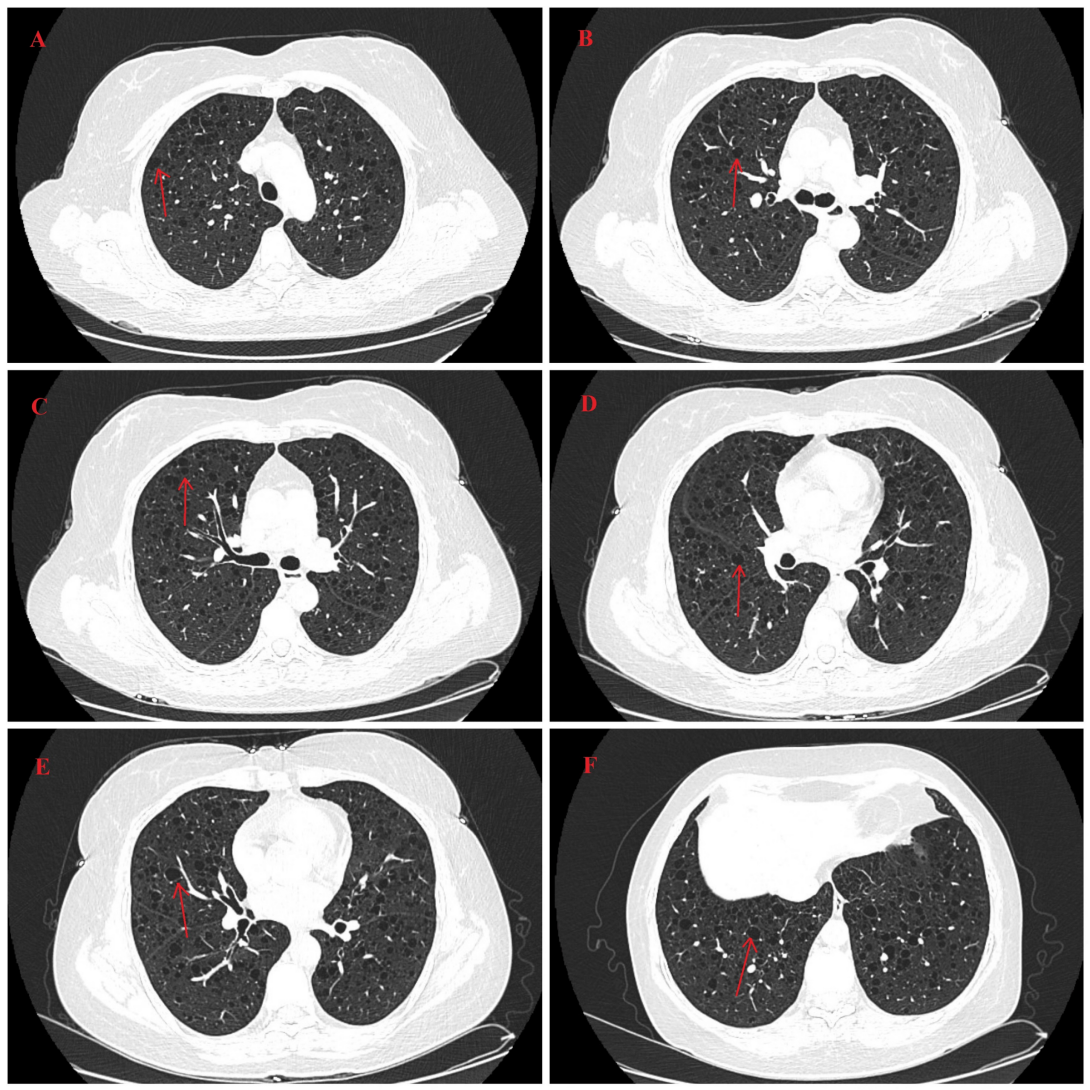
Lung CT Images (March 2025) (A–F) HRCT of the lung demonstrates diffuse emphysema and multiple cystic spaces in both lungs, suggestive of lymphocytic interstitial lung disease HRCT: high-resolution computed tomography
